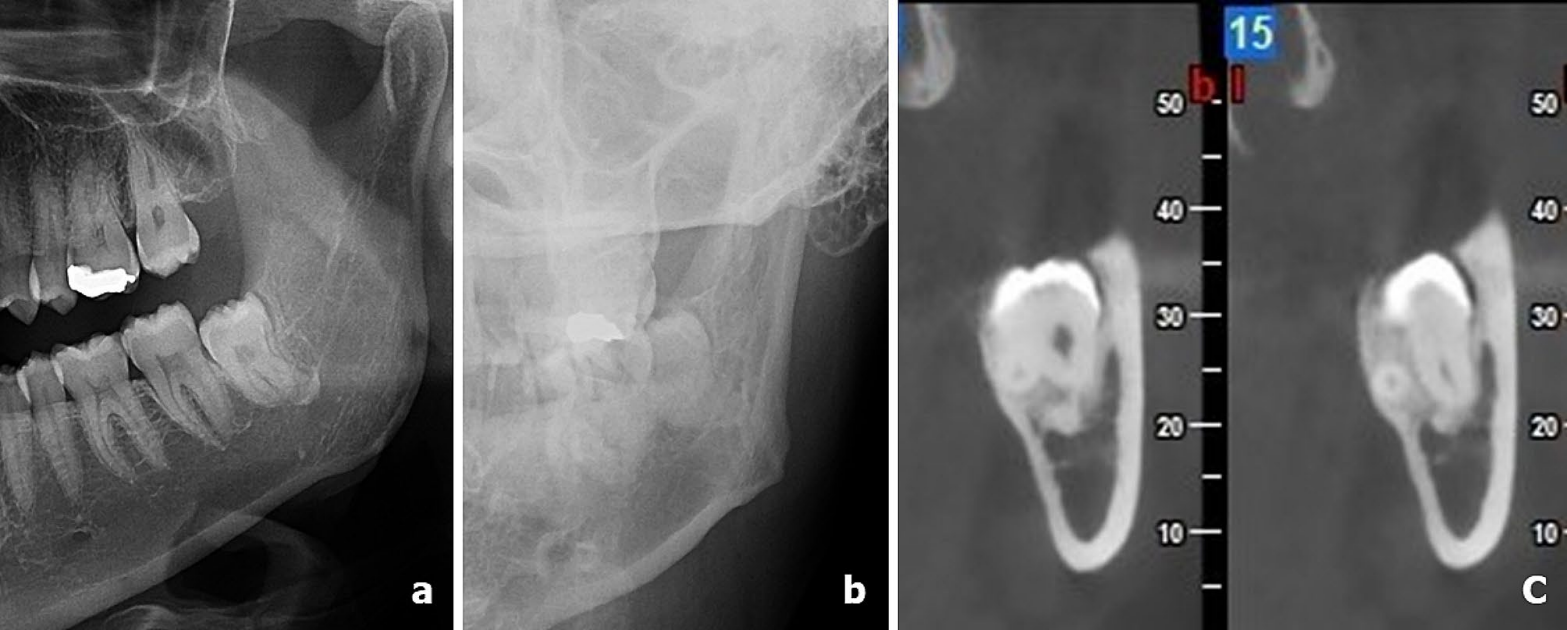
Cropped PR (a), cropped PA radiography (b), and cross sectional CBCT images (c) showing the presence of a relationship between MM3 roots and IAN
